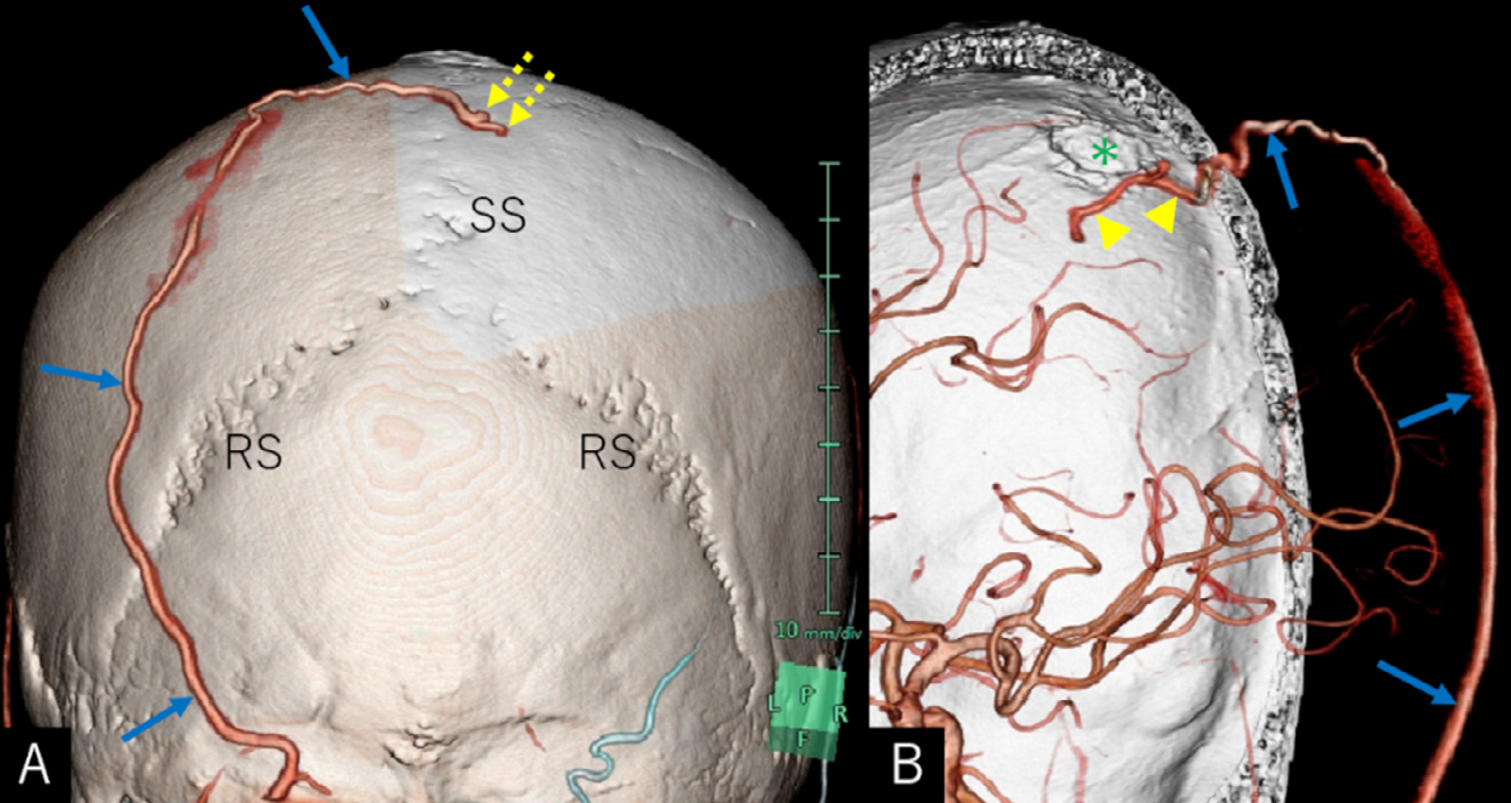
(*A*) Rear view of three-dimensional CT showing the ectatic left occipital artery (*arrows*) coursing upward, crossing the midline, entering into the right parietal foramina, and finally connecting with a dilated meningeal vessel that supplies the tumor (*dashed arrows*). (*B*) Interior view of the right parietal bone showing the occipital artery (*arrows*) that penetrates the parietal foramen and anastomoses with the meningeal vessel (*arrowhead*) adjacent to the bone erosion (*asterisk*) in the inner skull. RS: rhomboid suture; SS: sagittal suture.
